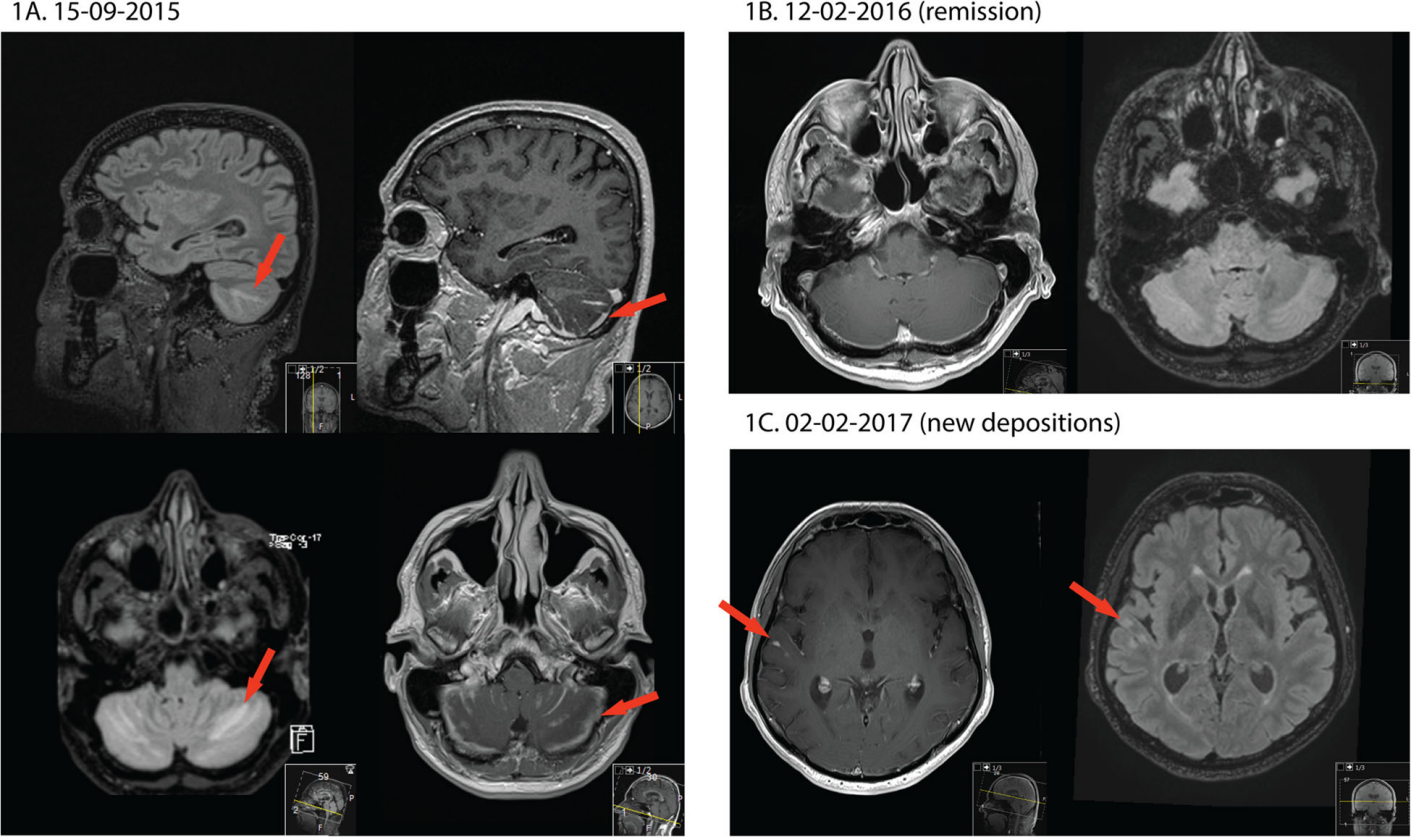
MRI FLAIR Imaging reveals cerebellar oedema. a: initial presentation. Sagittal (upper) and transversal images. FLAIR (left images) reveal parenchymal edema, while T1 post-contrast (right images) shows extensive leptomeningeal enhancement. b: normalization of MRI findings. c: New nodular leptomeningeal enhancement with adjacent limited parenchymal edema. Examples of radiological findings are indicated with red arrows
